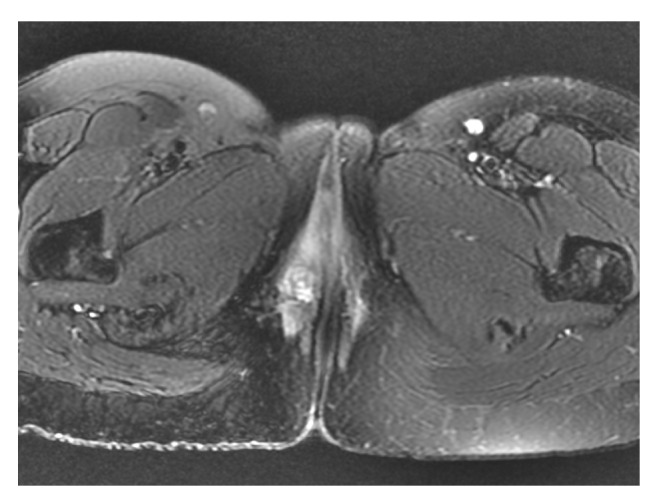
Preoperative T2 axial MRI though the pelvis demonstrating ACCBG lesion in the right vulva.
